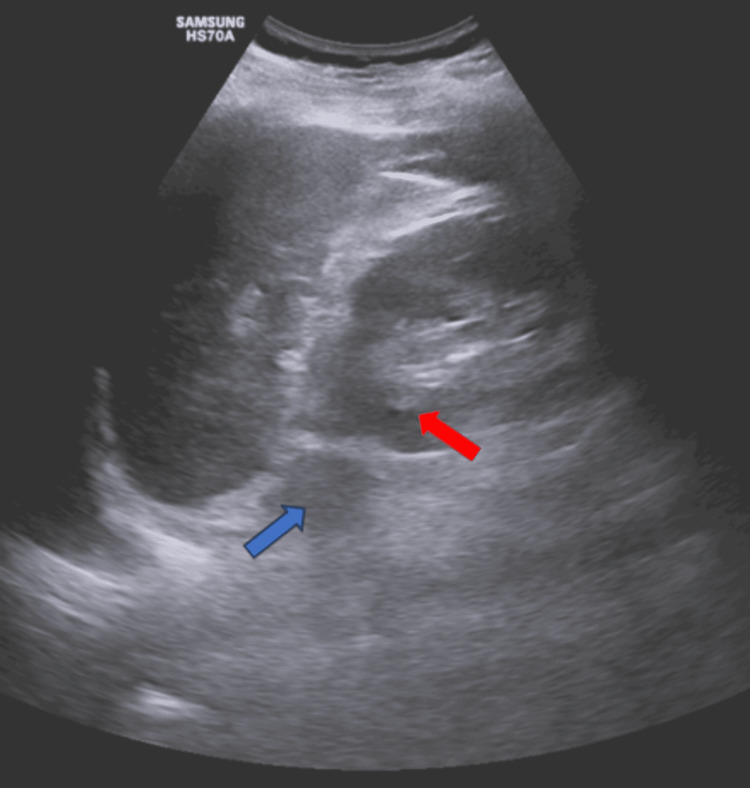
Ultrasonography of the abdomen and pelvis This figure shows a solid mass lesion in the left adrenal gland (blue arrow), which is iso-echoic to the left renal cortex (red arrow)
